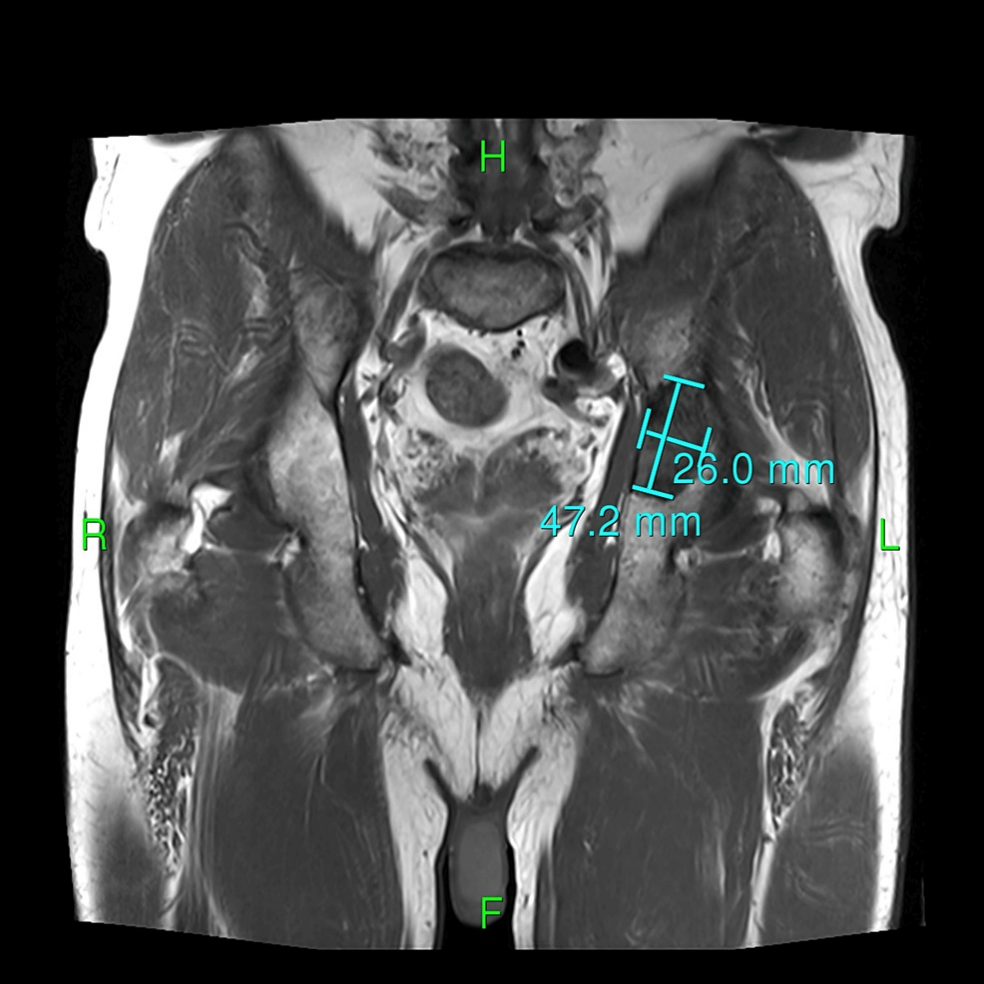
MRI coronal view of the left hip acetabulum tumor MRI: magnetic resonance imaging
